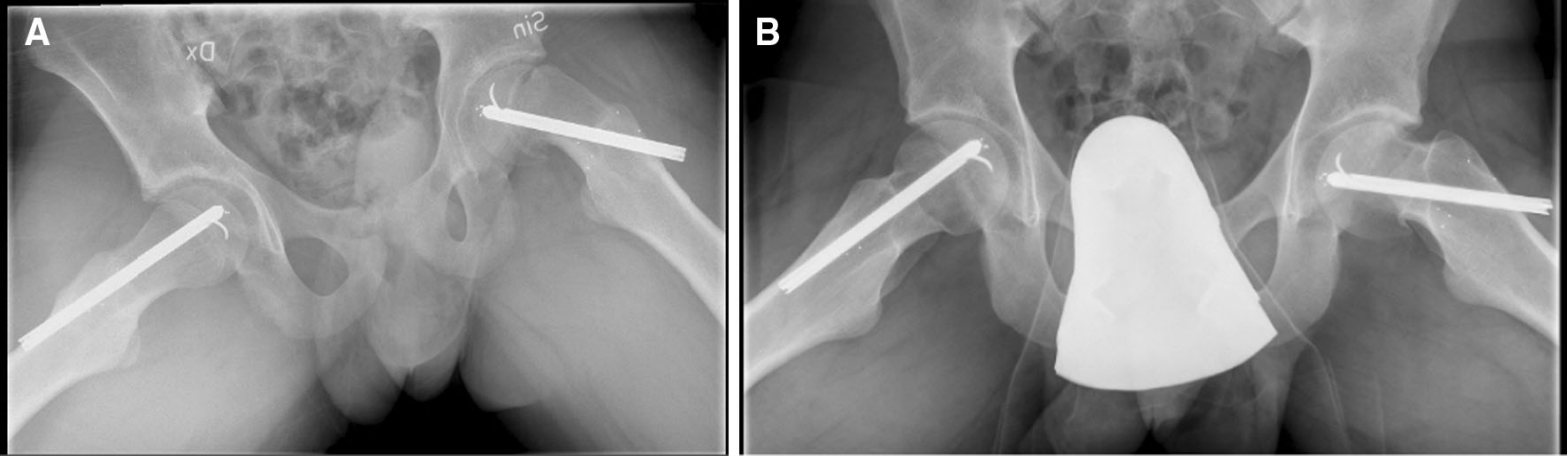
Radiograph immediately postoperative (a) and 3.4 years later (b), at skeletal maturity. Continued growth and remodelling can be detected, but it is difficult to define and quantify
